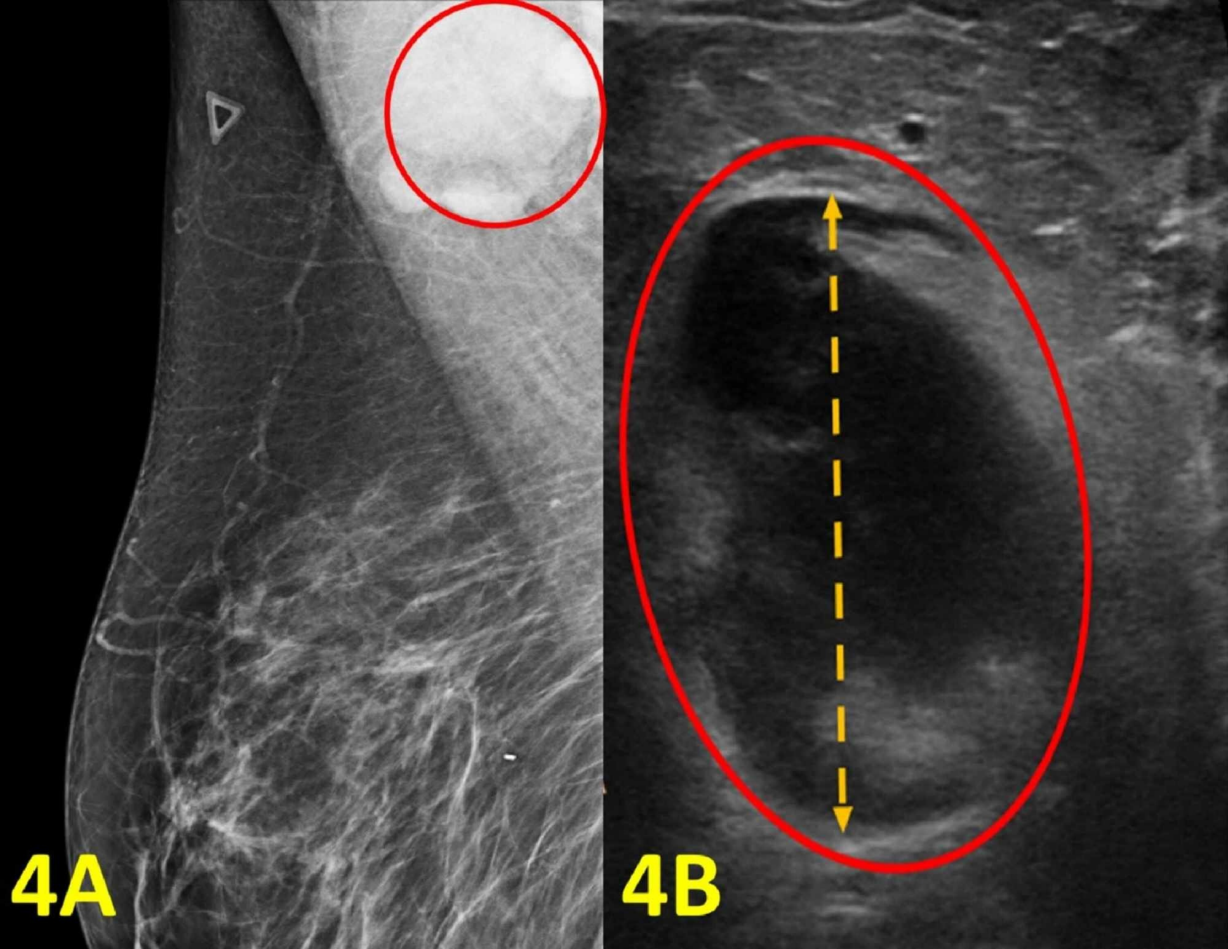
Imaging of the Right Breast and Right Axilla (A) Right breast diagnostic mammogram shows an enlarged lymph node in the right axilla (red circle). (B) Right axilla diagnostic ultrasound shows an enlarged axillary lymph node with cortical thickening (red oval) measuring 31 mm (orange double arrow).
